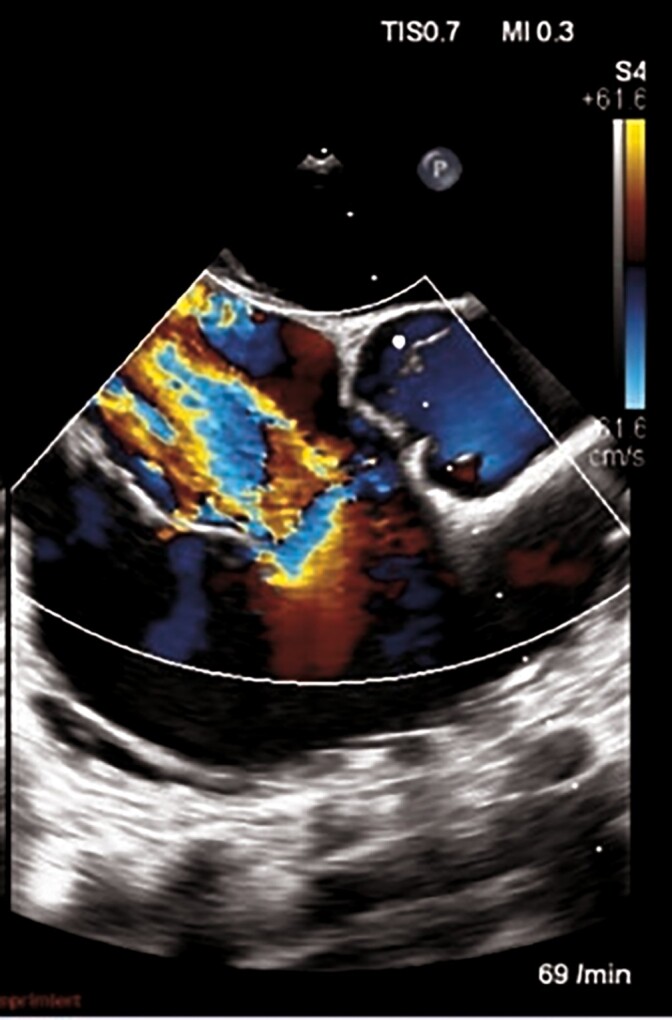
Intraoperative transesophageal echocardiography. Evidence of anterior leaflet tricuspid valve prolapse with rupture of primary chordae.
